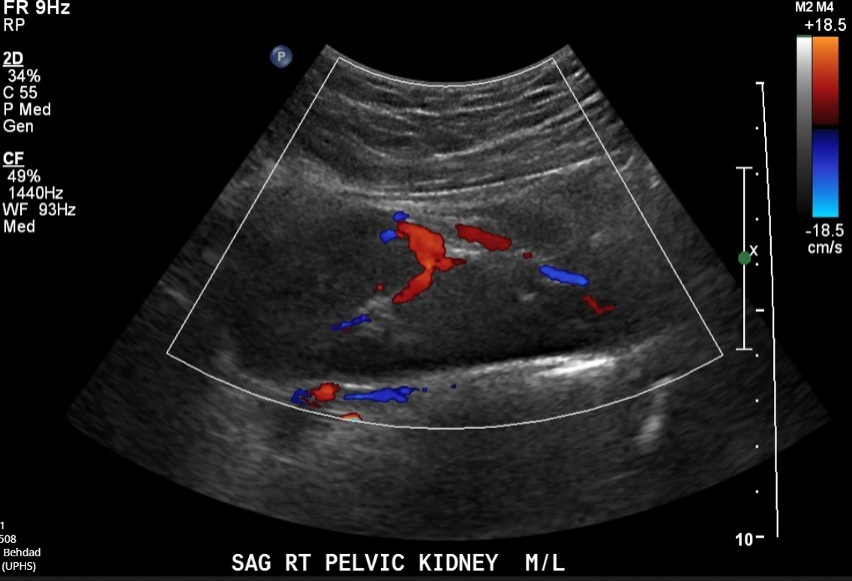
Doppler mode oblique imaging plane of right iliac fossa from case 2 demonstrating the presence of patent renal vasculature within the renal parenchyma in this ectopic location.
